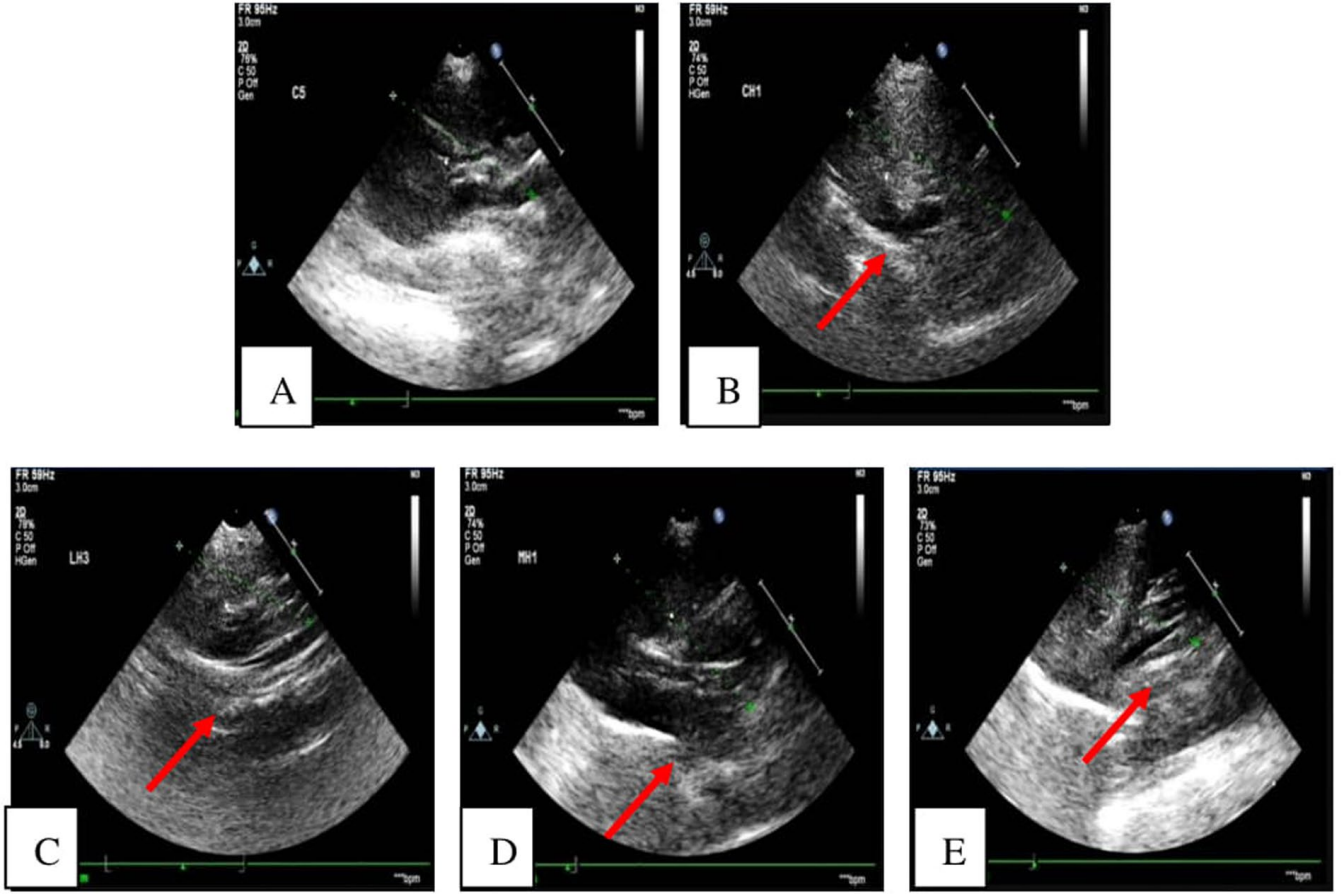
Ultrasound imaging of Liver. (A) C-ND group; (B) C-HSHFD group; (C) NP-L-HSHFD group; (D) NP-M-HSHFD group; E: NP-H-HSHFD group.
